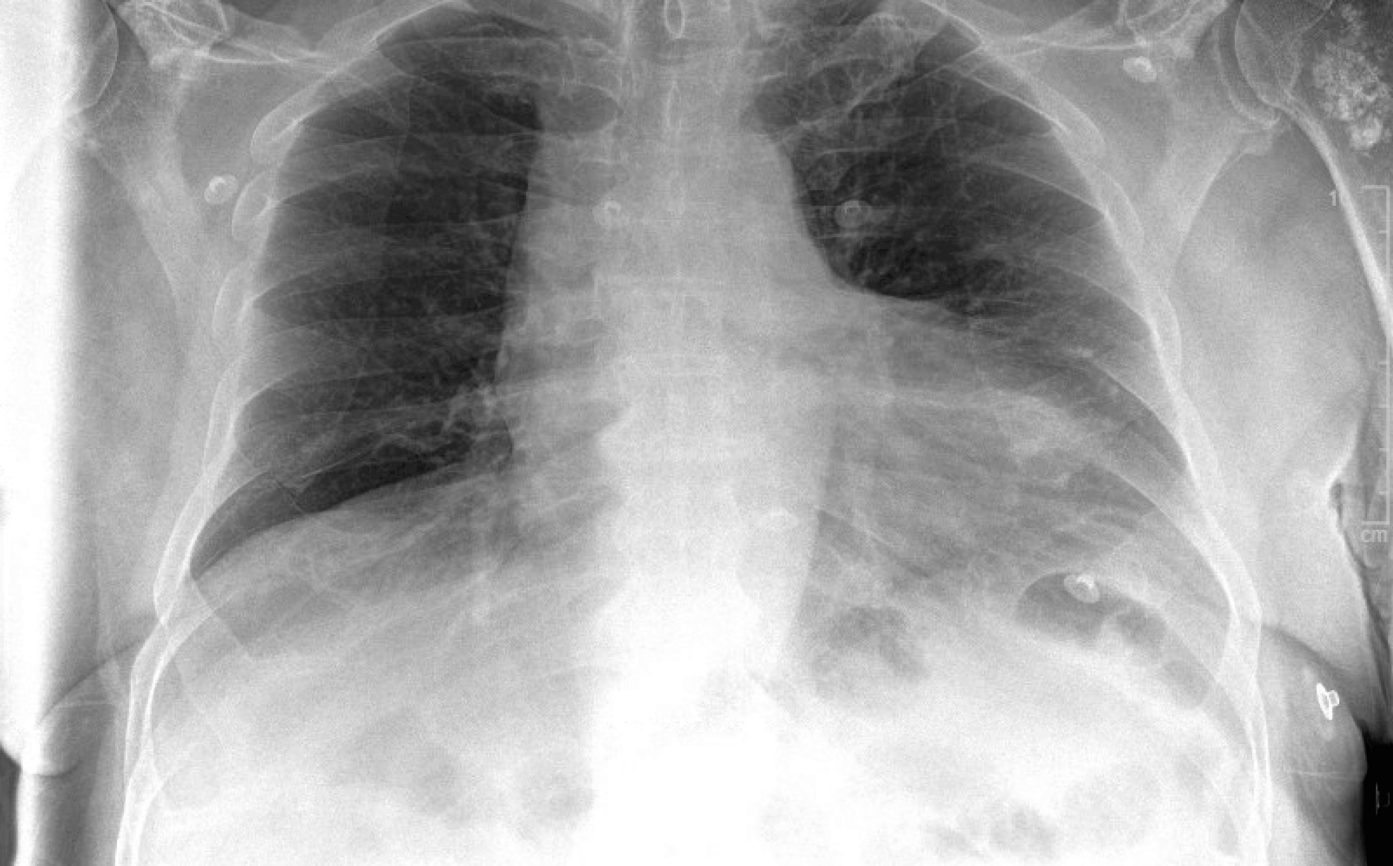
Chest X-ray showing no acute cardiopulmonary process; the cardiomediastinal silhouette is unchanged compared to the prior study.
